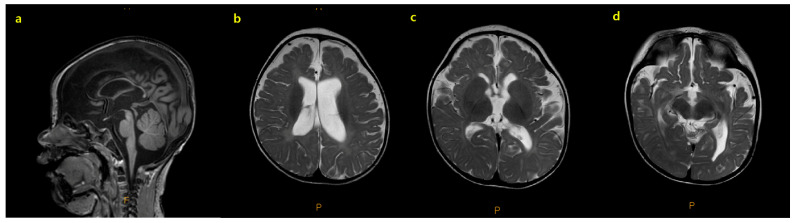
Brain magnetic resonance image(MRI) findings of the patient. Brain MRI findings of the patient. Dysmorphic thin corpus callosum was observed in the T1 weighted sagittal image (a). The diffuse cerebral atrophy, characterized by prominent extra axial space, prominent cerebral sulci with decreased volume, and delayed myelination of white matter were also evident (b–d).
